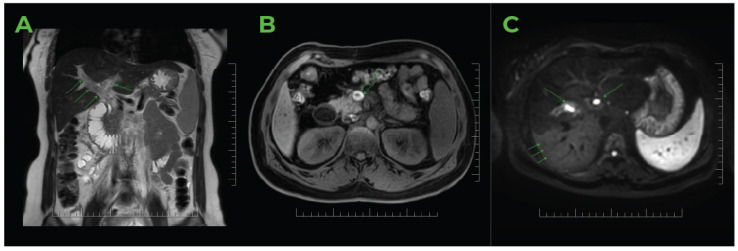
MRI study of the abdomen of pregnant women in the 14th week of gestation. (A) The coronal plane T2 demonstrated an abnormal hyperintense signal in the dilated lumen of the portal vein, affecting the branches to the right and left liver lobes. The absence of a flow void signal indicates vein thrombosis, with accompanying findings of splenomegaly. (B) The axial plane T1 exhibited a pathological hyperintense signal in the enlarged lumen of the superior mesenteric vein, confirming the presence of a thrombus. (C) The axial plane DWI highlighted the thrombus in the branches of the portal vein in the liver (S8/S5, S2/3), as well as a difference in signal intensity within the parenchyma of the right lobe (S7/S6), confirming uneven vascular perfusion. *Source*: MJ.
